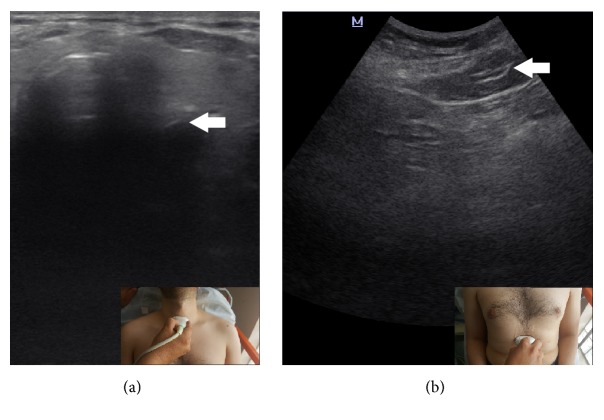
Neck ultrasound (a) and abdomen ultrasound (b) images show the nasogastric localizations. Small images denote the probe orientation.
